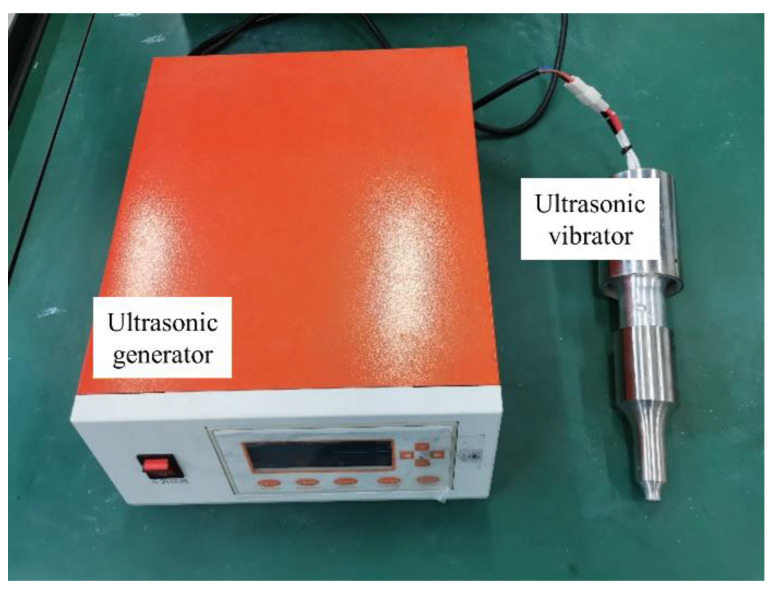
Ultrasonic generator and ultrasonic vibrator.
